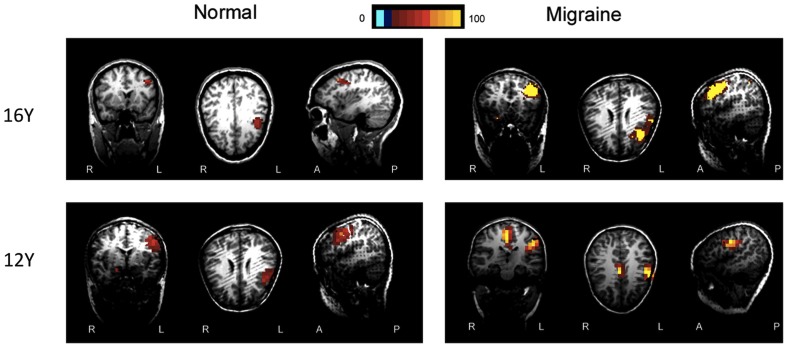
Magnetic source activation of high-gamma oscillations elicited by right finger movement. Magnetic Source Imaging (MSI), the combination of magnetoencephalography (MEG) results and magnetic resonance imaging (MRI), shows the source activation elicited by right finger movement in both children with migraine and healthy controls. The red and yellow areas indicate regions of neuromagnetic activation (or synchronized neural firing). The neuromagnetic activation elicited by right finger movement is localized in the contralateral motor cortex in healthy controls (“Normal”). The neuromagnetic activation elicited by right finger movement is localized in the contralateral motor cortex as well as the premotor and supplementary motor areas in children with migraine (“Migraine”).
